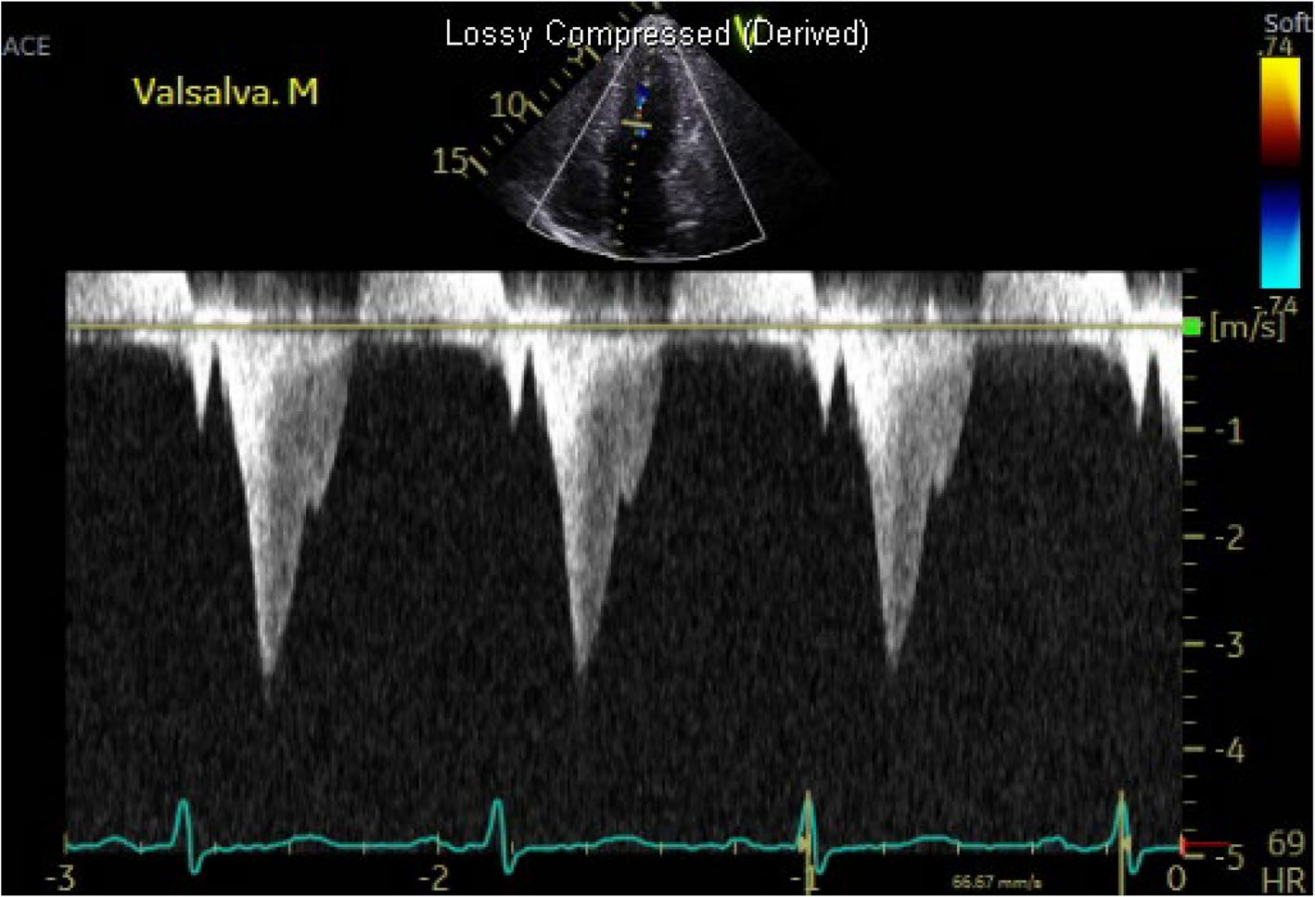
Continuous-wave Doppler demonstrates a relatively early-peaking velocity profile
